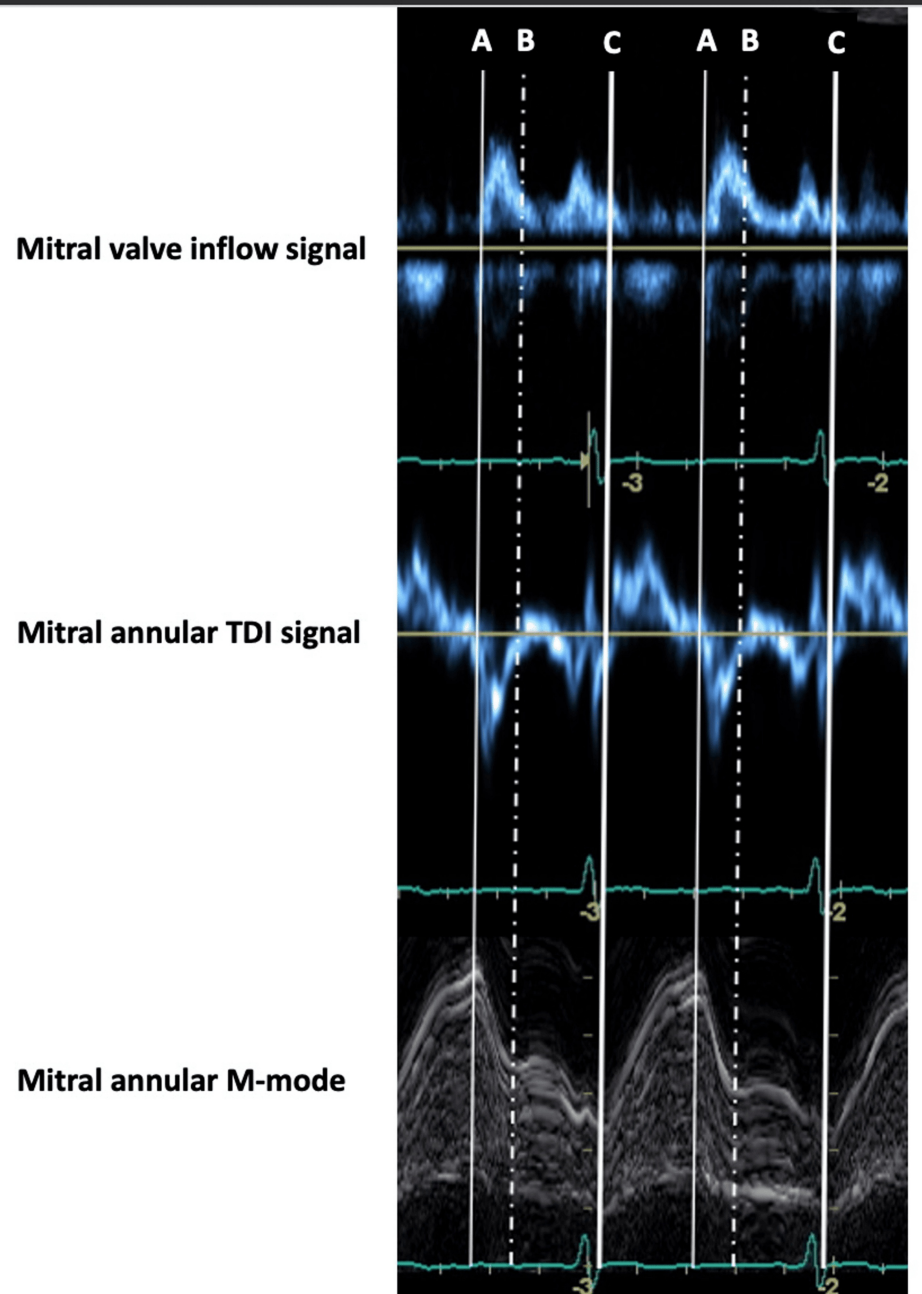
Representative images of mitral inflow, mitral annular TDI, and M-mode from the same patient, showing the correlation among cardiac events as they are individually depicted in each imaging modality. Two cardiac cycles are shown. (A) Thin white line indicates the onset of LV diastole, (B) dashed white line corresponds to the end of early LV diastole, and (C) thick white line identifies the onset of LV systole. TDI, tissue Doppler imaging; LV, left ventricle.
